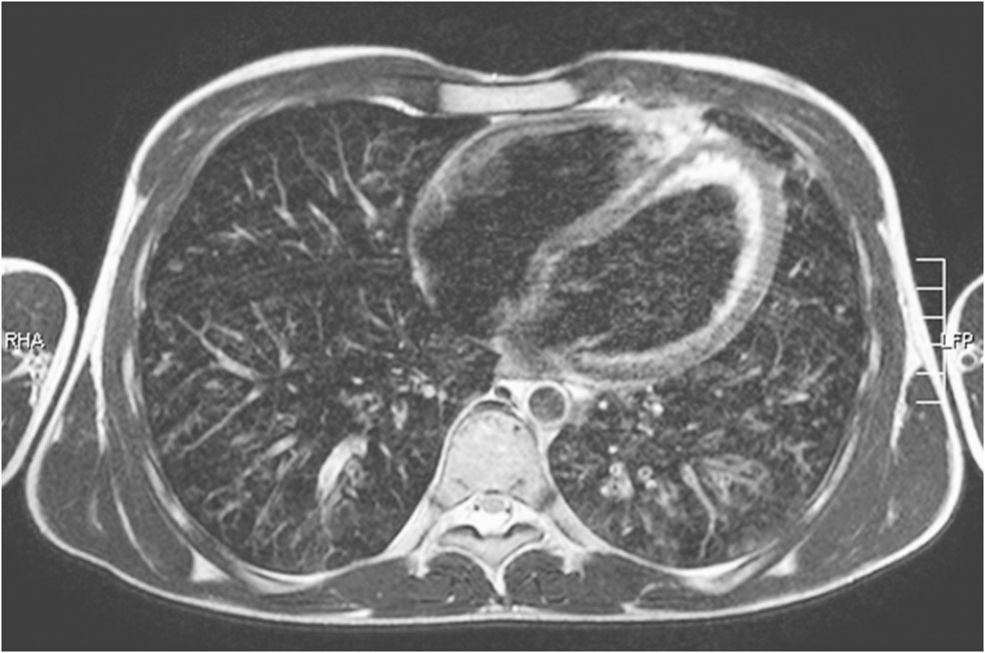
Normal, good-quality lung MR in a 9-year-old girl (axial T2-weighted turbo spin-echo respiratory-triggered examination, parameters in Table 1). The arteries and bronchi are of equal width and have a dark lumen as well as a delicate signal-rich wall. The veins are predominantly bright, their walls indistinct. There is no relevant cardiac or breathing artifact
